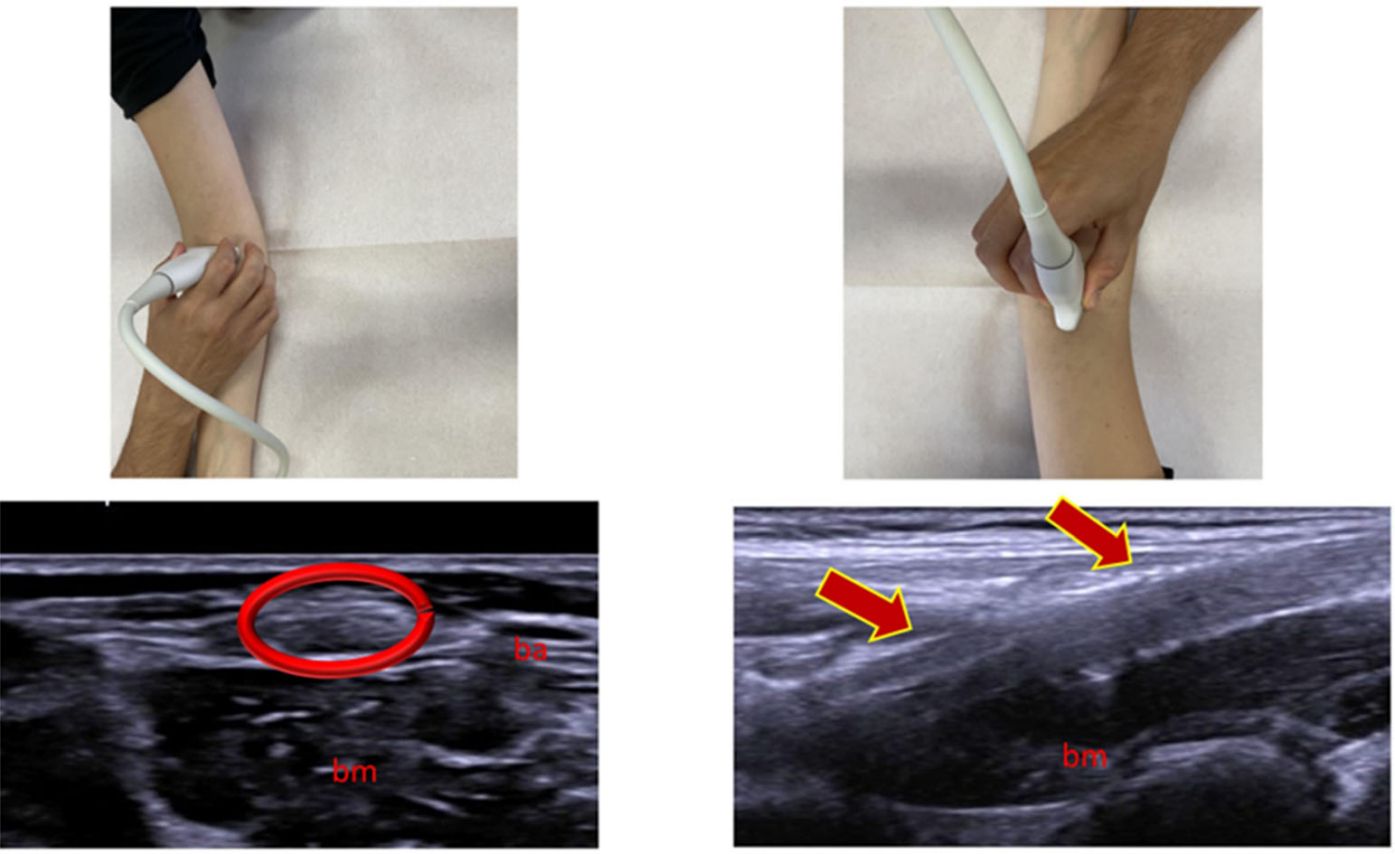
Ultrasound image acquisition points and corresponding anatomical structures. Top row: Photographs showing the positioning of the ultrasound probe for scanning the distal biceps brachii tendon (left) and the distal triceps brachii tendon (right). Bottom row: Corresponding ultrasound images of the tendons. The left image (circled in red) shows the biceps brachii tendon (ba) and brachialis muscle (bm). The right image (indicated by arrows) shows the triceps brachii tendon (bm: Brachialis muscle).
